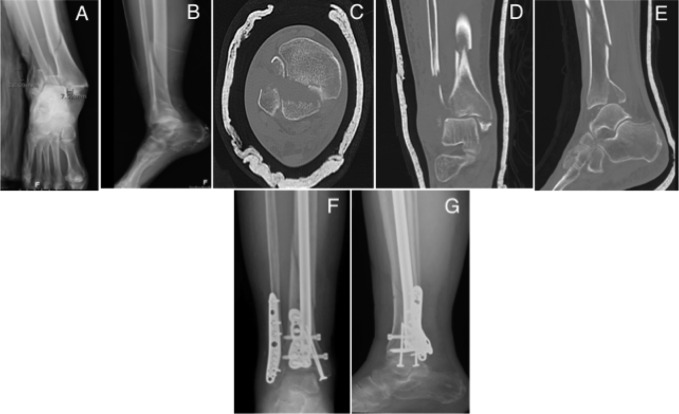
Radiograph demonstrating the (A) AP and (B) lateral injuries of a distal tibial fracture with intra-articular extension into the plafond. CT images (C) axial (D) coronal and (E) sagittal of the injury. Radiographs (F) AP and (G) lateral at 6 weeks postoperative fixation with IMN and plate fixation of intra-articular fracture with maintained alignment.
